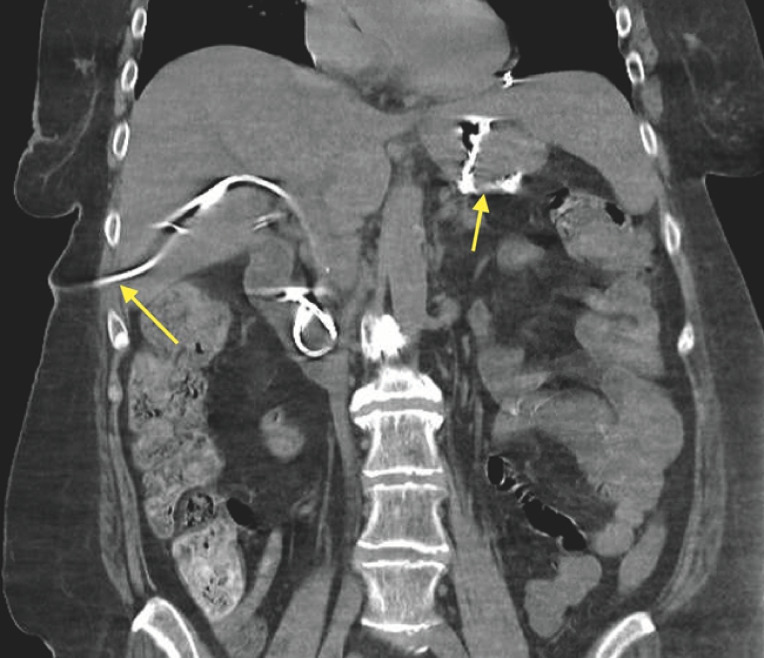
Computed tomography showing successful placement of a percutaneous biliary drain and gastric pouch (arrows).
